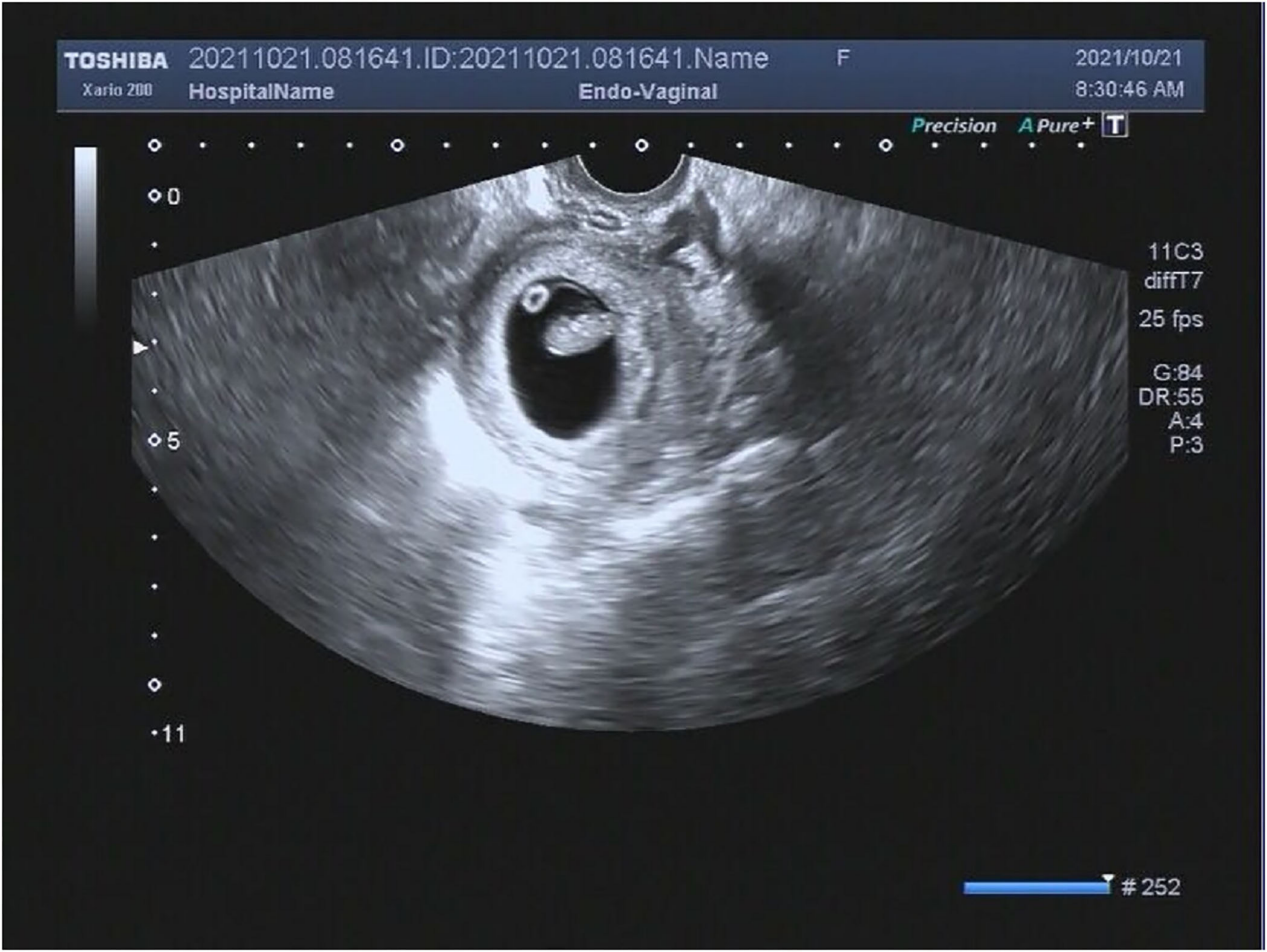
Transvaginal sonography of 8 weeks of gestation of pregnancy.
